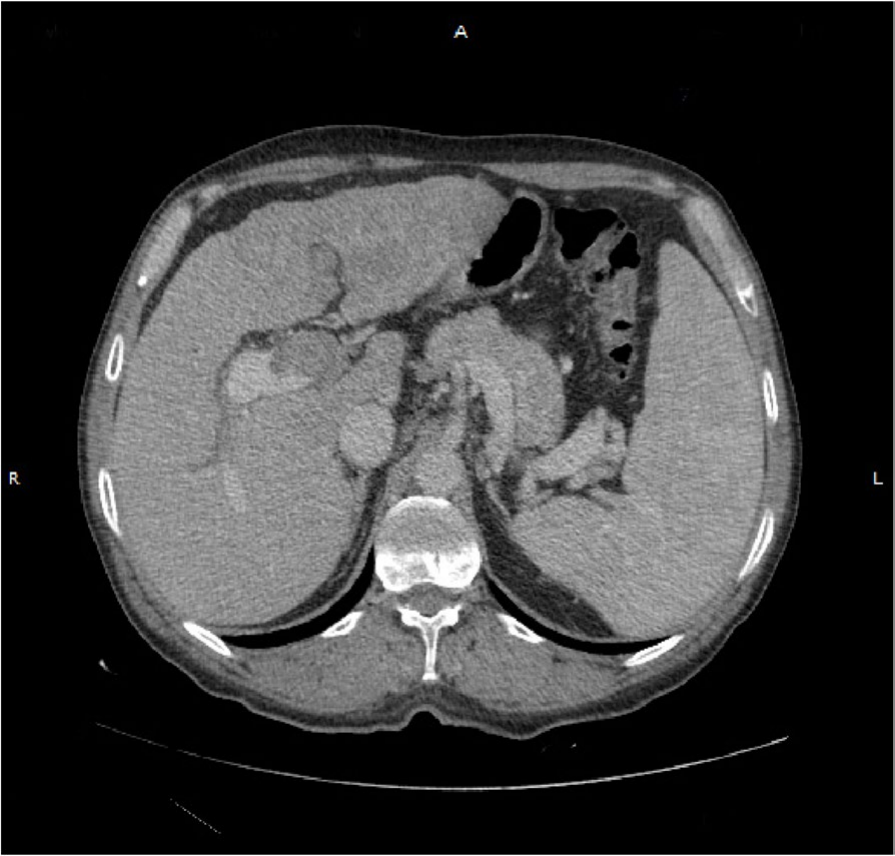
Portal phase of CT abdomen showing washout with infiltration of portal vein, with near total thrombus, which appears the same in the delayed phase
